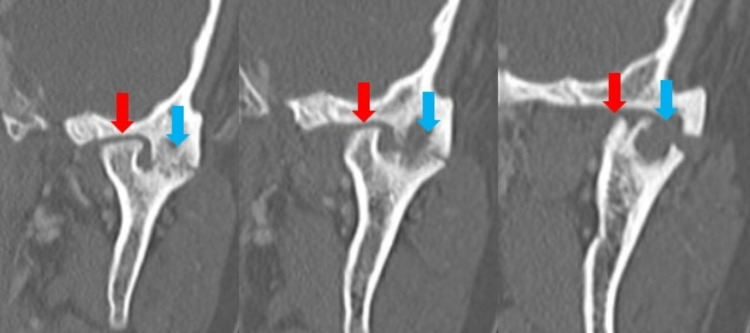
CT images of the left TMJ in coronal view. Flattened medial articulation (red arrow) and lateral articulation with osseous bridging and erosive changes (blue arrow) of bifid mandibular condyle. TMJ: Temporomandibular joint.
